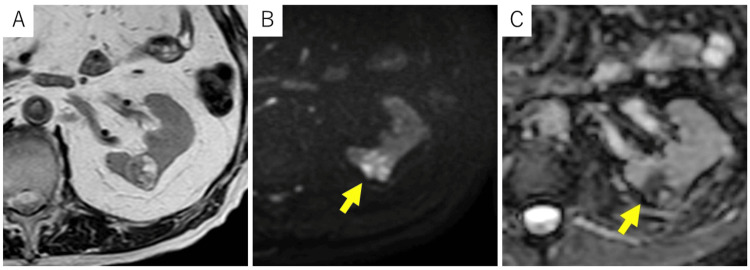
MRI of the abdomen A round mass was identified in the mid-portion of the left kidney. On T2-weighted imaging (A), the lesion showed a mixture of intermediate and high signal intensity areas. It was clearly demarcated from the surrounding normal renal parenchyma by a low-signal rim suggestive of a pseudocapsule. DWI (B) demonstrated diffusely high signal intensity throughout the lesion, while the ADC map (C) demonstrated a heterogeneous pattern with both low and high signal areas ADC: apparent diffusion coefficient; DWI: diffusion-weighted imaging; MRI: magnetic resonance imaging
